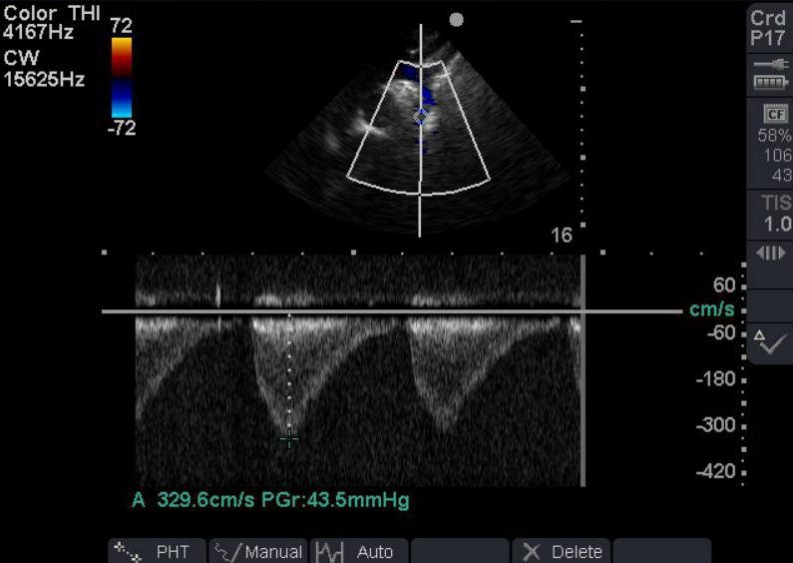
Abnormal continuous Doppler flow pattern in proximal descending aorta from suprasternal notch consistent with coarctation physiology.
